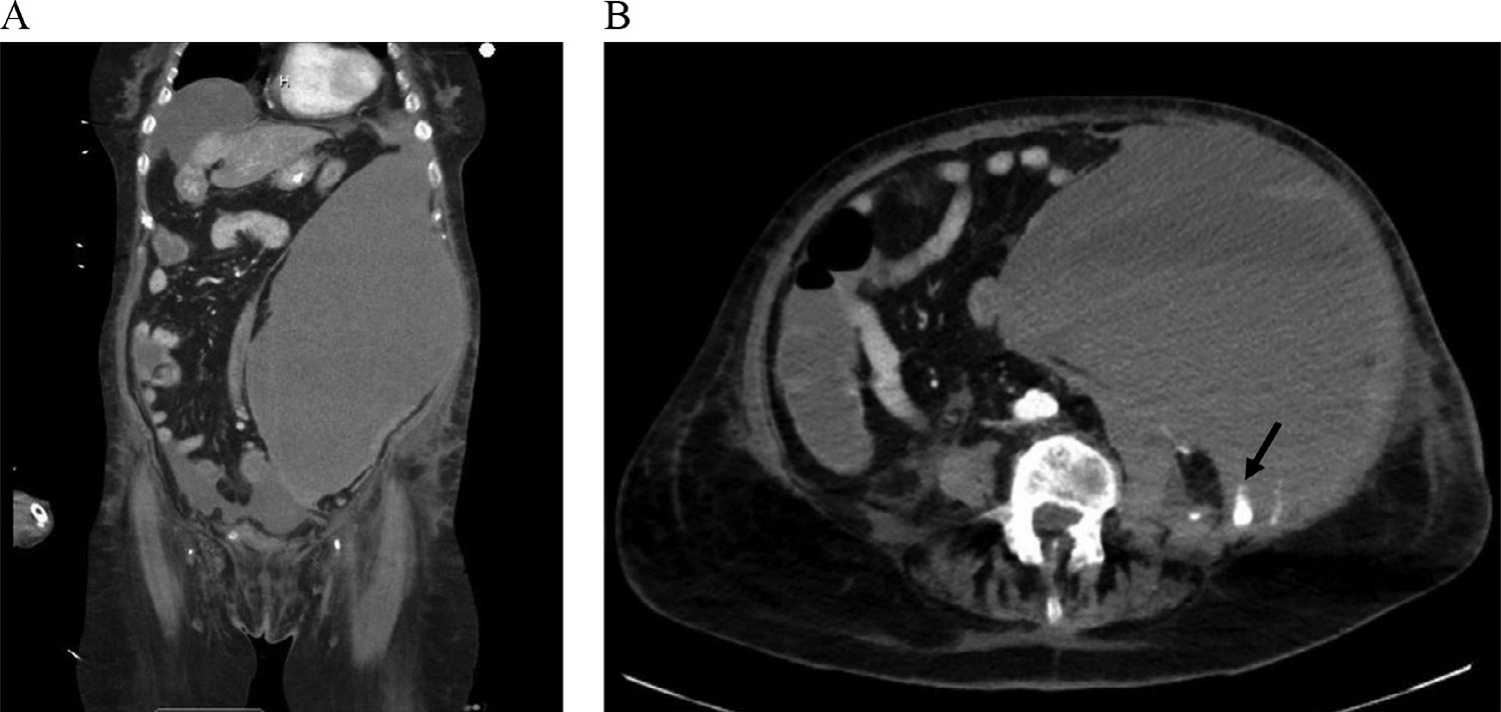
(A) Coronal CT Angiography image through abdomen demonstrates a very large retroperitoneal hematoma. (B) Axial CT Angiography image through the mid abdomen demonstrates a large blush of active extravasation of contrast as well as several smaller blushes within the hematoma.
